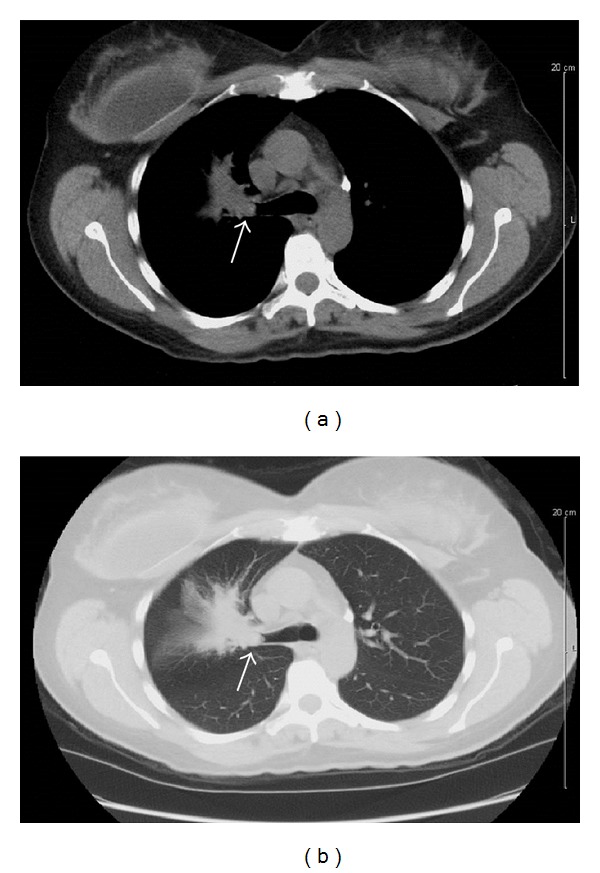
Computerized tomography (CT) of the chest showing a mass (arrow) measuring 1.5–2 cm obstructing the right upper bronchus with associated atelectasis. No evidence of metastatic disease is seen. (a) Mediastinal window. (b) Lung window.
